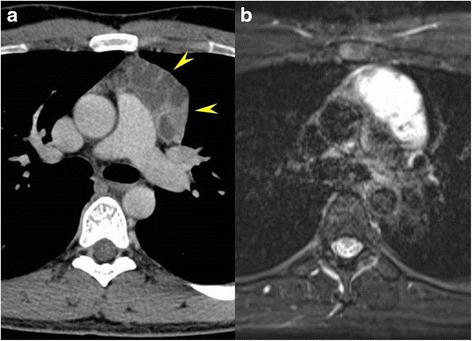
a Chest CT showing an anterior mediastinal tumor (6.3 cm × 3.6 cm × 9.0 cm, *arrowheads*) with multilocular cystic changes. b Fat-saturated T2-weighted MRI showing cystic lesions. No apparent invasion to the adjacent structures
